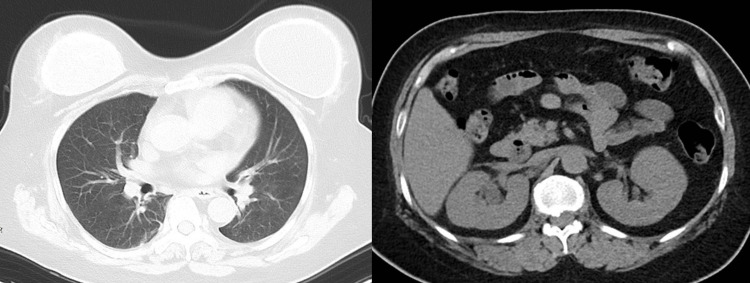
CT images obtained at admission Chest CT (left) and abdominal CT (right) showed no apparent abnormalities.
